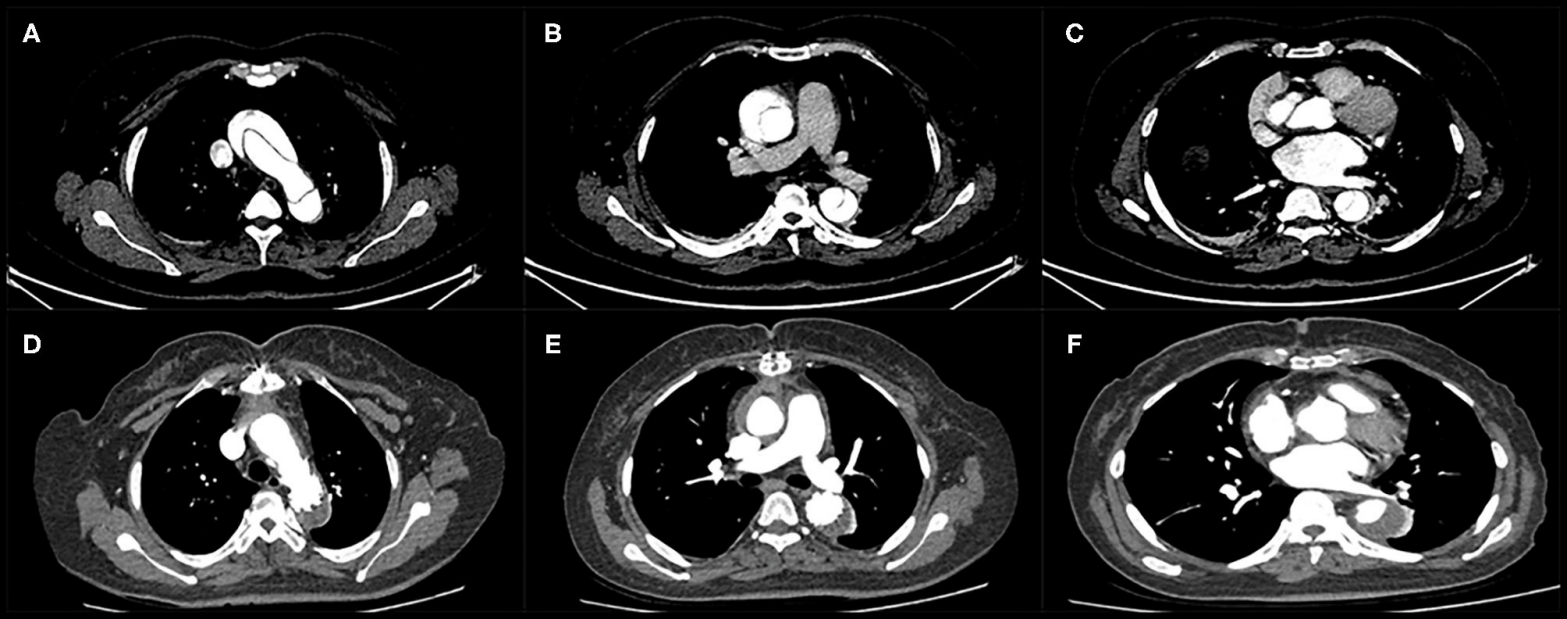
Computed tomography angiography of a patient with acute type A dissection before surgery (A–C) and during follow-up (D–F). Thrombus formation in the false lumen was observed around the stented graft and distal to the edge of the stented graft.
